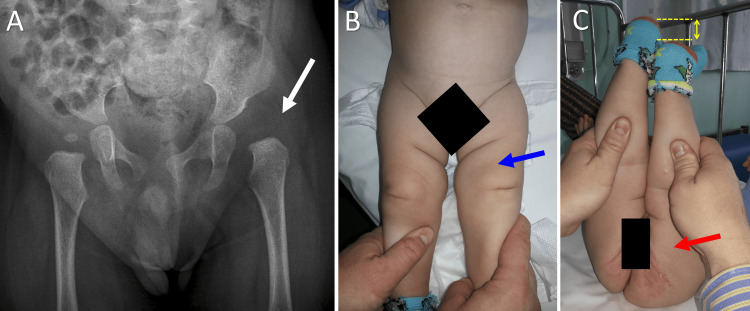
No asymmetric skin fold (ASF) formation seen in a six-month-old infant with a left Ortolani hip A. An anteroposterior pelvic view of the X-ray indicating dislocation of the left hip (white arrow); B. No ASF is detected on the left thigh or groin (blue arrow); C. Proximal migration of the left femur is evident, leading to a shorter left thigh (yellow arrow, yellow lines). No ASFs are evident on the left gluteal region as well (red arrow). Image credits: P. Samelis
